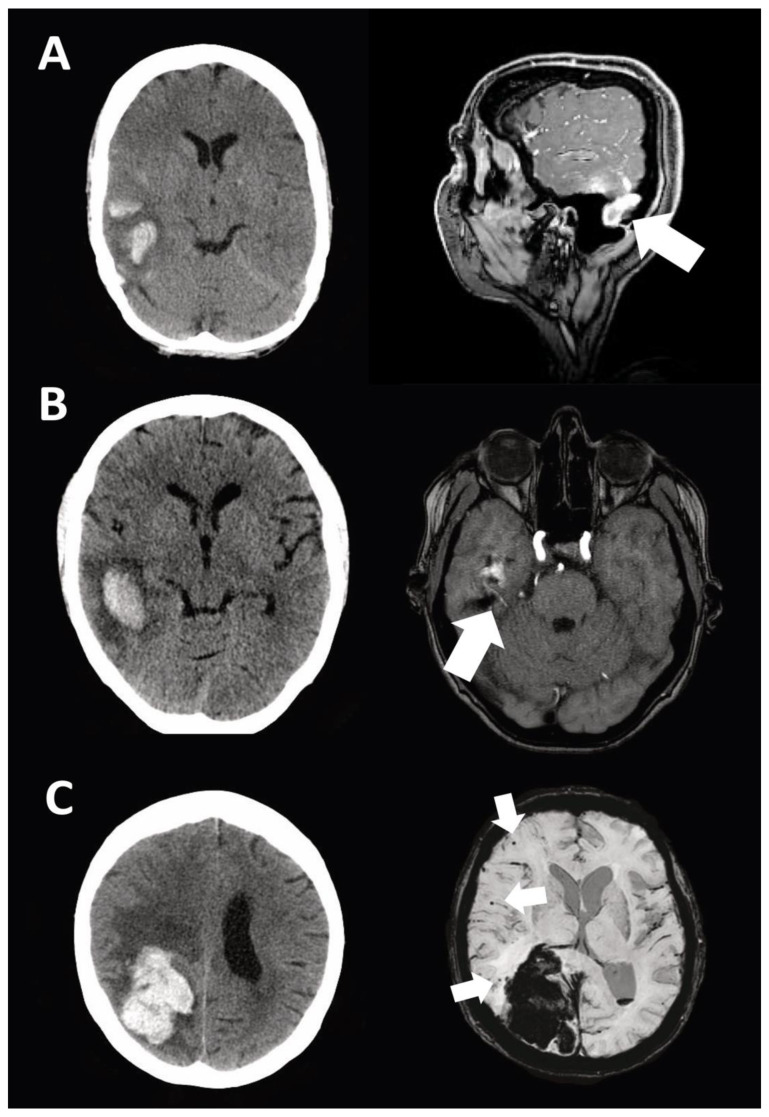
Exemplary cases with magnetic resonance imaging work-up for lobar ICH. (A) Lobar ICH caused by sinus venous thrombosis; (B) Lobar ICH caused by dural arteriovenous fistula; (C) Lobar ICH accompanied with underlying cerebral amyloid angiopathy (CAA). White arrow indicates the described pathology.
